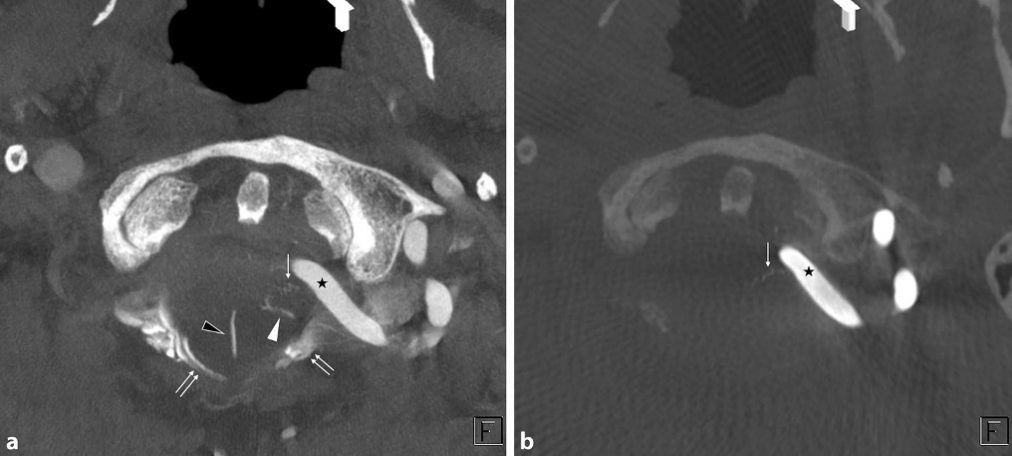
Differentiation between arteries and veins in contrast-enhanced cone-beam CT (CE-CBCT). The axial view of the slab MIP images of CE-CBCT (a) and arterial phase three-dimensional rotational angiography (b). CE-CBCT with long acquisition mode includes data on arteries and veins. We can only observe arteries (*arrow*) in arterial phase three-dimensional rotational angiography (b). Consequently, we can confirm that the other vessels are veins (*white and black arrowheads*) or sinuses (*double arrows*) in CE-CBCT (a). *Star* shows the left vertebral artery
